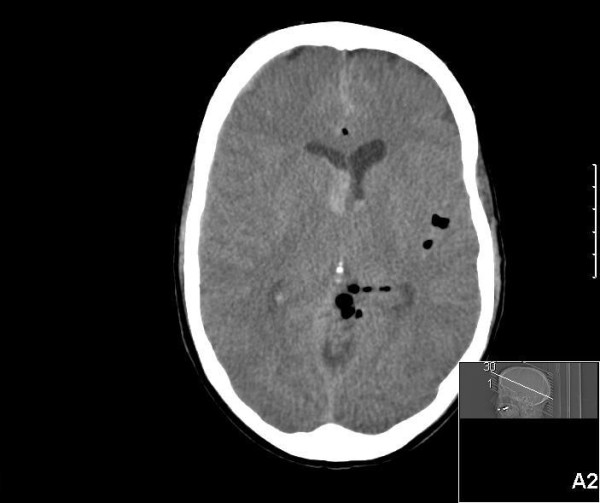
CT showing a subarachnoid and intraventricular hemorrhage and pneumocephalus.
